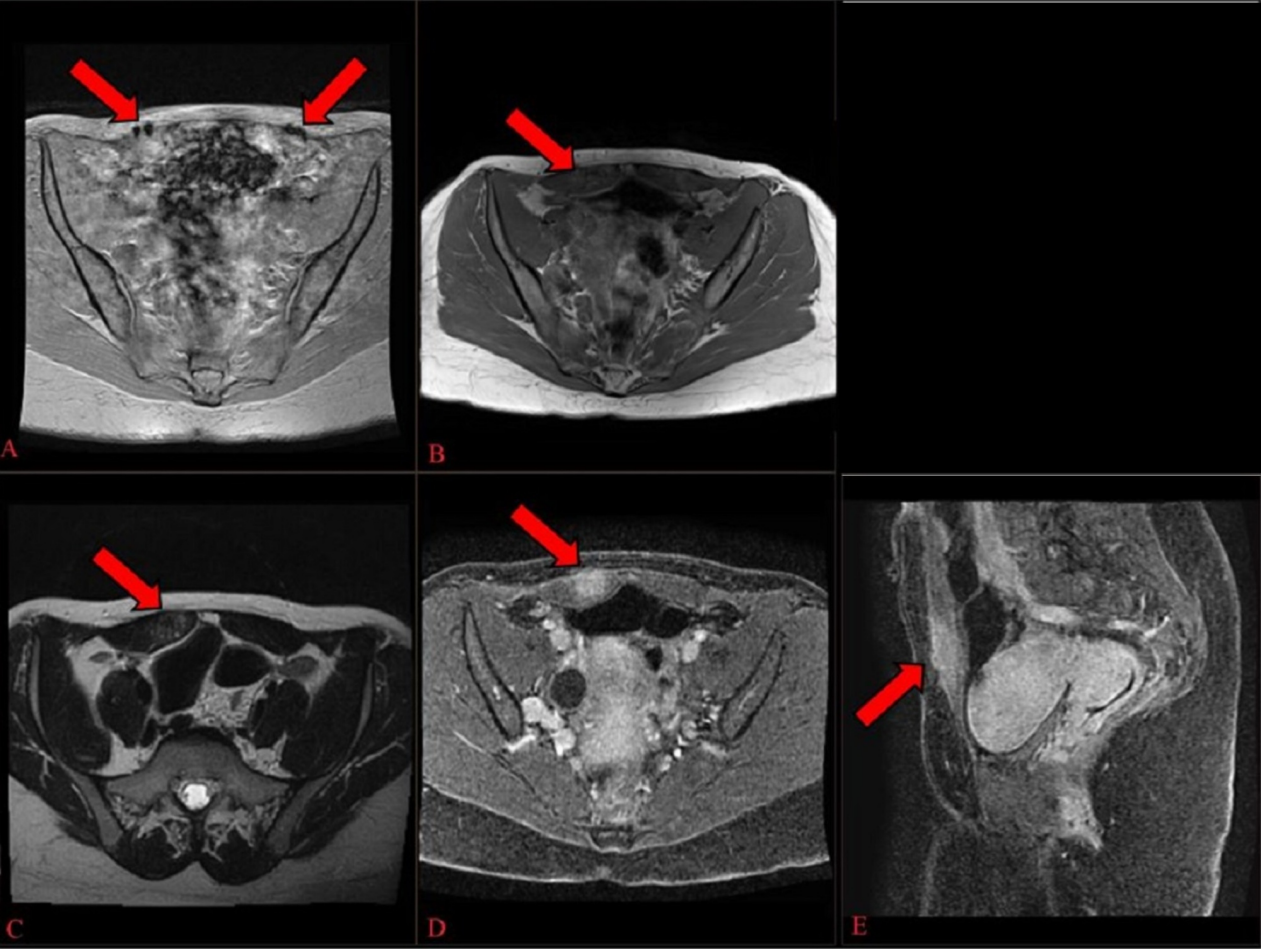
Magnetic resonance imaging of the pelvis A) Gradient image demonstrates "blooming" artifact overlying the rectus abdominis muscle corresponding to patient's low transverse cesarean section incision site; B, C) T1 and T2-weighted axial unenhanced images demonstrate a heterogenous mass in the right rectus abdominis musculature; D, E) Post-contrast T1-weighted gradient echo images demonstrate enhancement of the mass.
